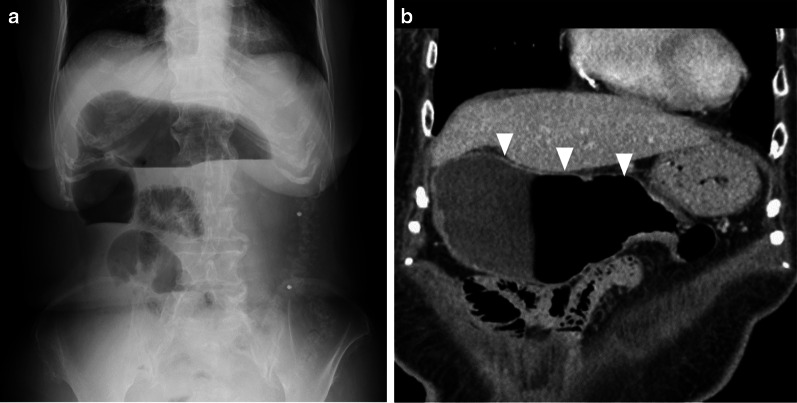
Abdominal X-ray and contrast-enhanced computed tomography (CT). a X-ray shows massive colon gas niveau formation in the epigastric region. b Dilation of the cecum, ascending colon, and transverse colon proximal to the splenic flexure seen on the contrast-enhanced CT scan. No mechanical obstruction is present (arrowhead)
